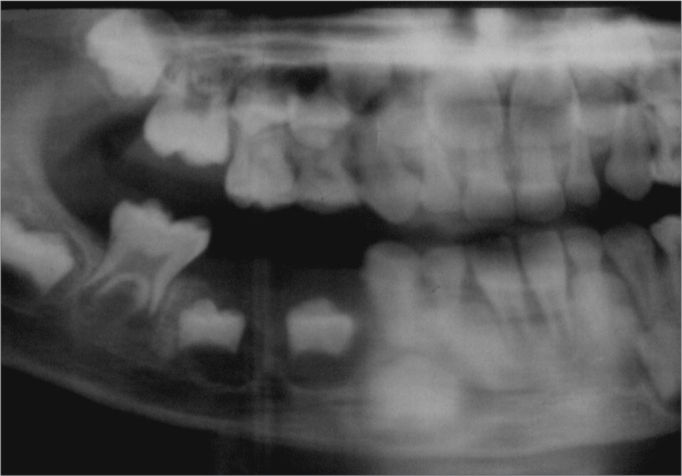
Burkitt's lymphoma - Panoramic radiograph - a diffuse translucid area is seen on the mandible premolar area.
